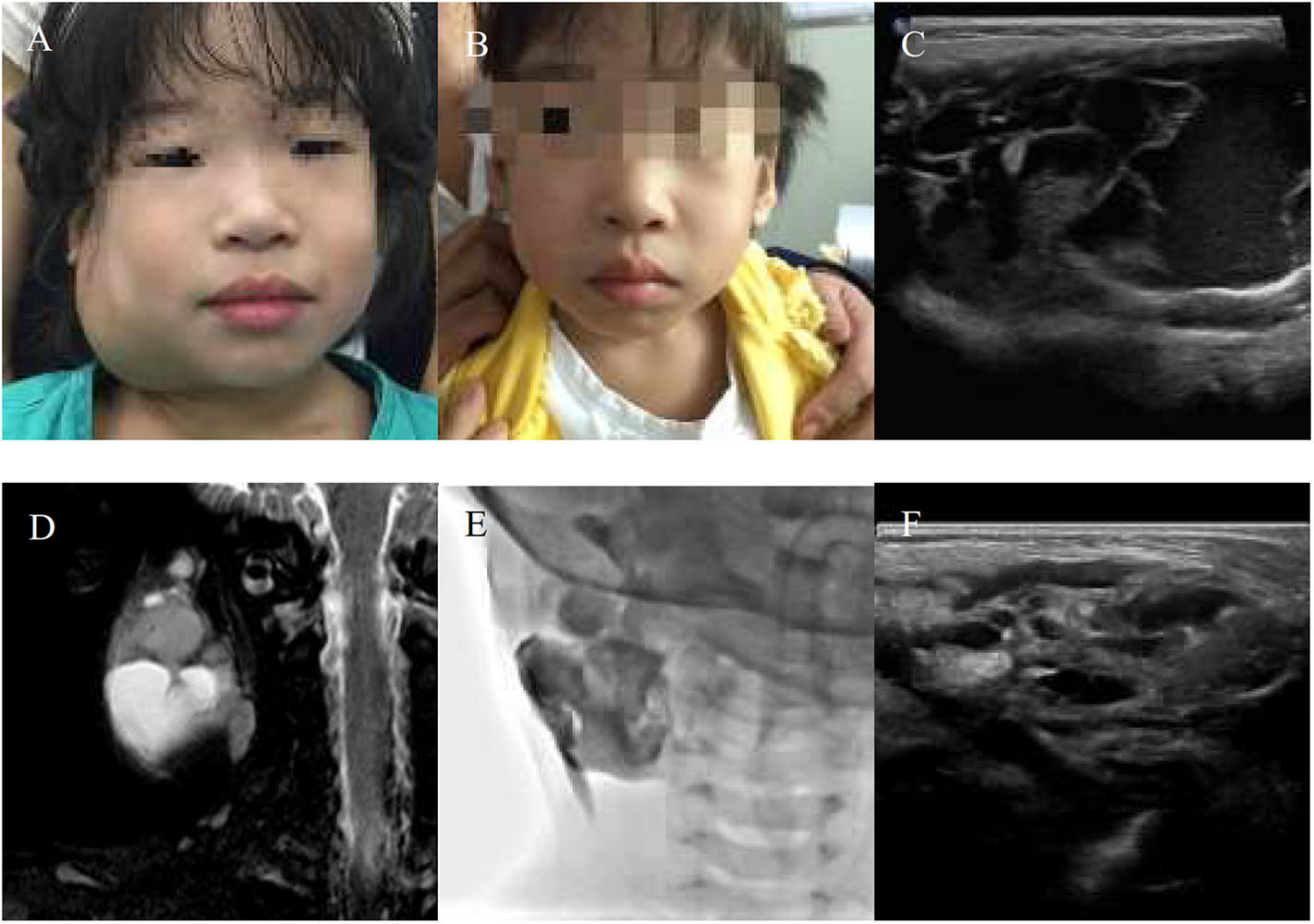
A 10-year-girl with combined lymphatic malformation (LMs) in the right parotid region gets excellent resolution (A–F). (A) Before treatment. (B) After bleomycin injections after one injection. (C,D) Some radiological features are sufficiently suggestive of diagnosis of LMs. (E) The lymphatic malformation filled with bleomycin-visipaque mixture. (F) Reexamin ultrasonography shown a few of the residual lesions.
